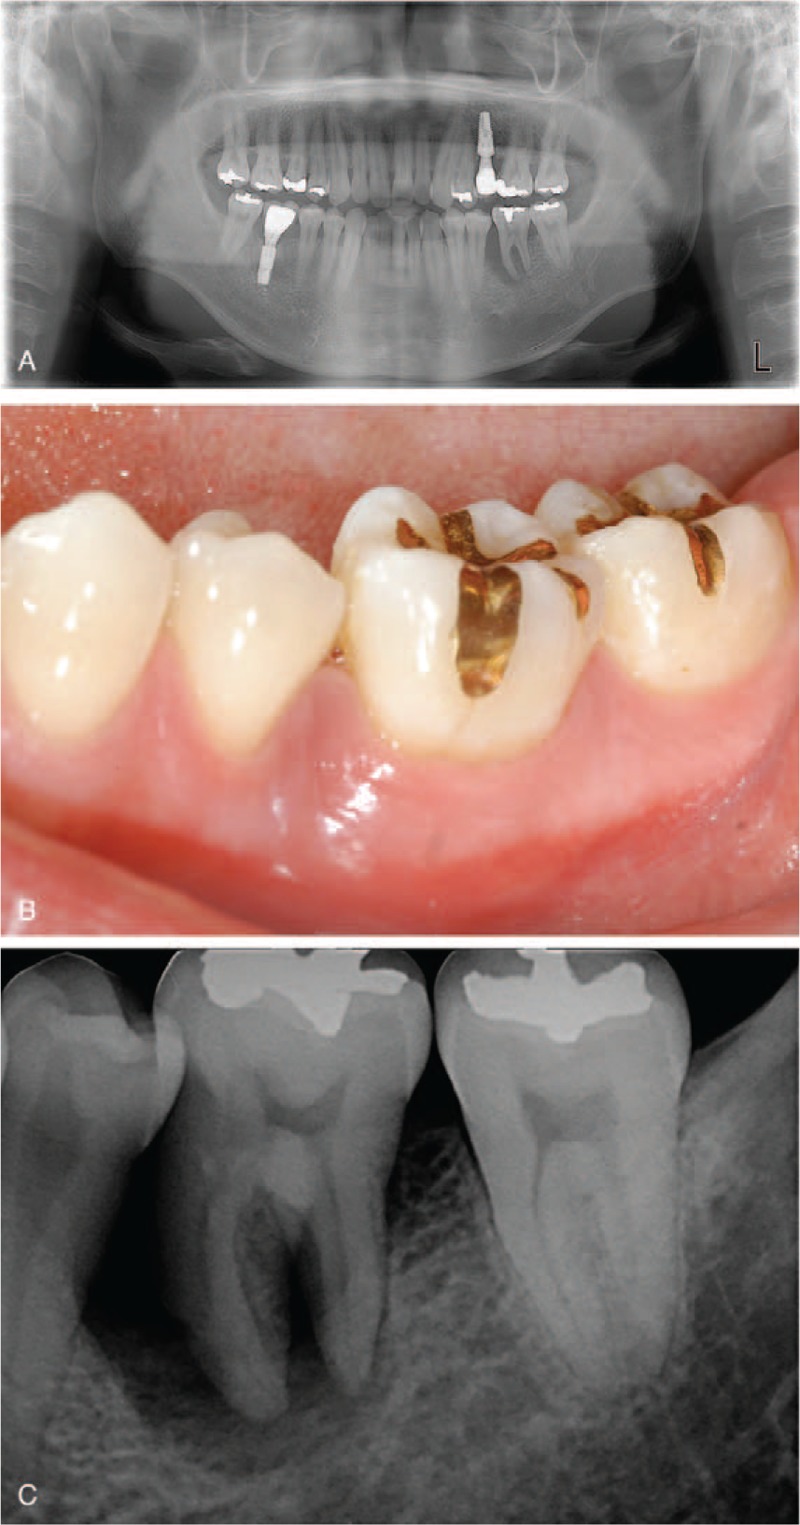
Evaluation of apically involved tooth. (A) Panoramic radiographic evaluation. (B) Buccal view showing gingival redness. (C) Periapical radiograph showing the loss of supporting bone around the tested tooth with apical involvement of the mesial root.
